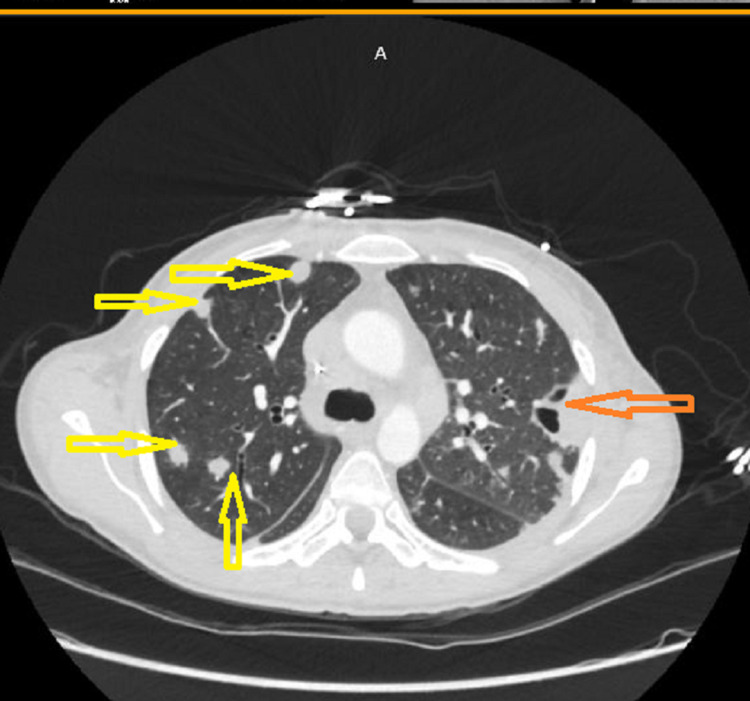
CT chest showing multiple septic pulmonary emboli (yellow arrows) and cavitary nodule (orange arrow) CT: computed tomography
